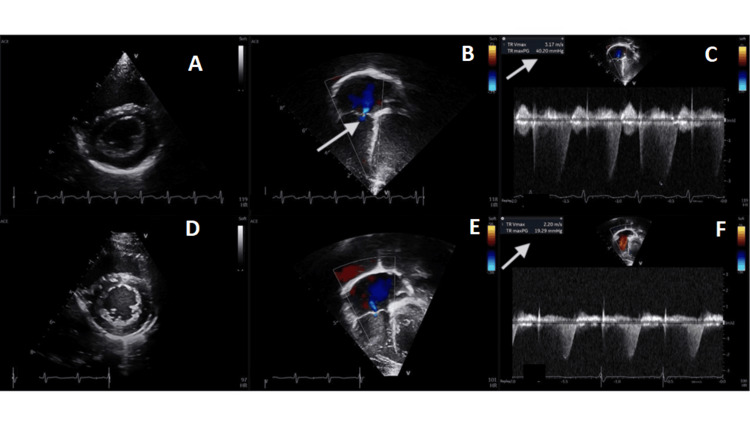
Echocardiographic comparison at different points of clinical course (A-C) Some loss of interventricular septum symmetry in A compared to D, normal right ventricular cavity, and trivial tricuspid valve insufficiency (arrow in B), with elevated tricuspid regurgitant velocity and tricuspid regurgitant pressure gradient (arrow in C). Features suggestive of PAH. (D-F) Normal right ventricular cavity, normal tricuspid valve, normal tricuspid regurgitant velocity, and normal tricuspid regurgitant pressure gradient (arrow in F). PAH: Pulmonary Arterial Hypertension
